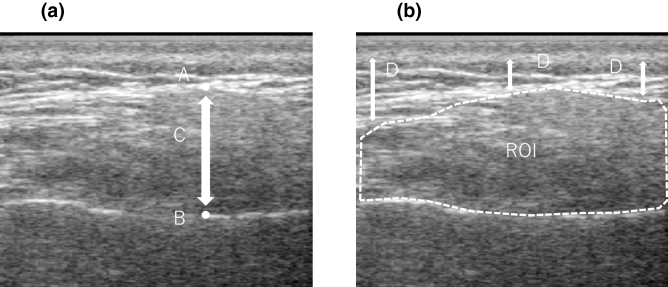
Masseter muscle evaluation by ultrasound diagnostic apparatus. (a) Masseter muscle thickness (MMT). (b) Masseter muscle echo intensity (MMEI) measurement. (A) Masseter muscle surface (B) Mandibular ramus (C) Masseter muscle thickness (D) subcutaneous fat thickness. *ROI* : region of interest.
